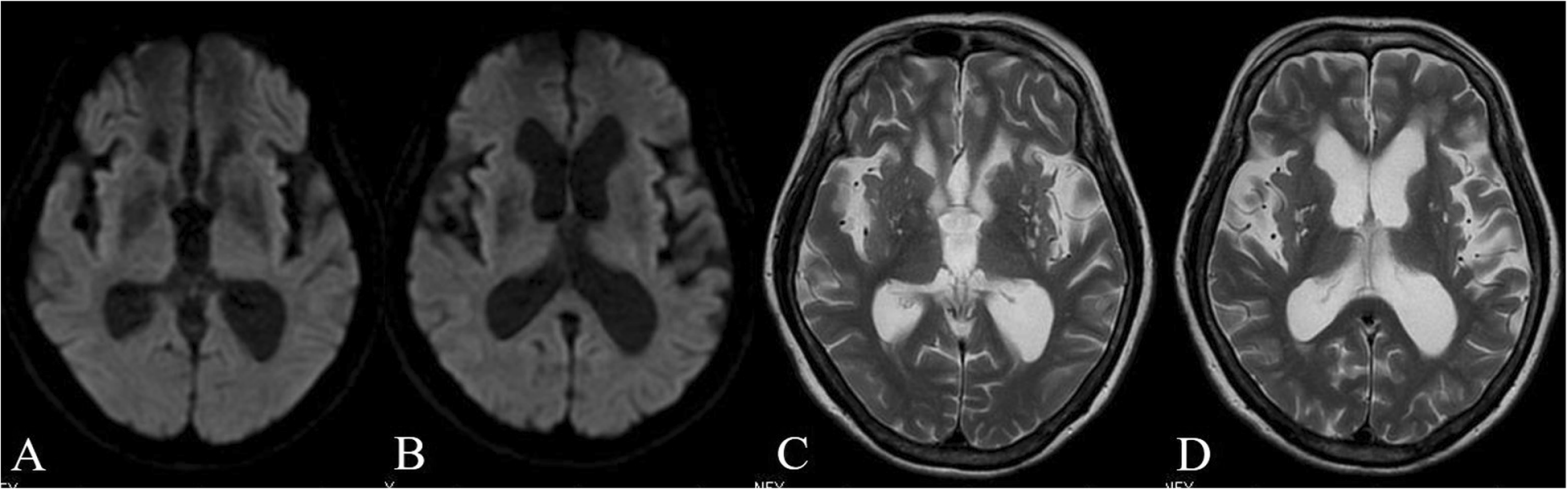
Follow-up magnetic resonance (MR) images on day 4. Diffusion-weighted MR image showing prominent regression of the hyperintense lesion (a, b). T2-weighted MR image showing small high-intensity spots in bilateral putamen due to old ischemic lesion, but no new abnormality was observed in the posterior limb of the left internal capsule (c, d)
